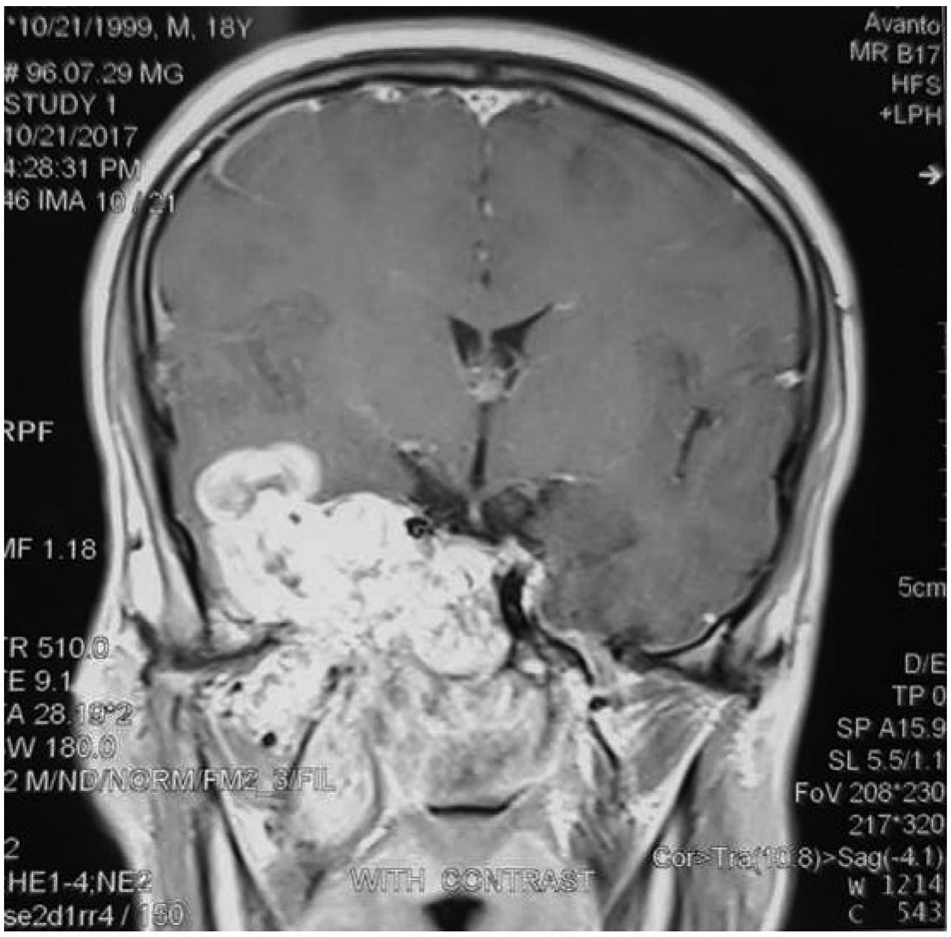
MRI showed a hypervascular mass with plenty of flow voids signal, due to containing large vessels, in the right nasal cavity with extension to intracranial and infratemporal spaces.
